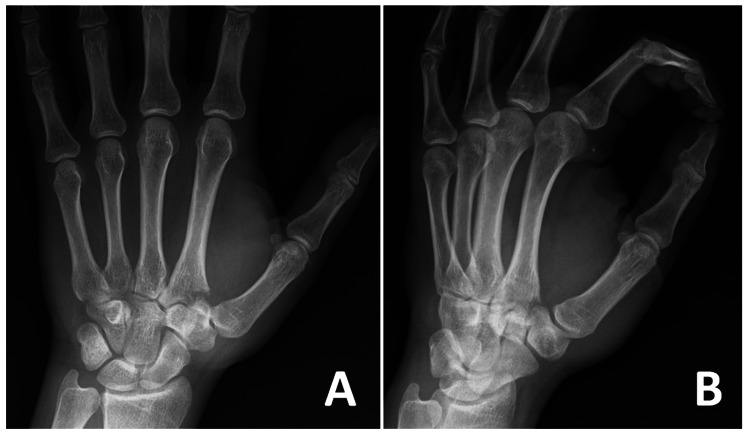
Preoperative (A) posteroanterior and (B) oblique hand radiographs
